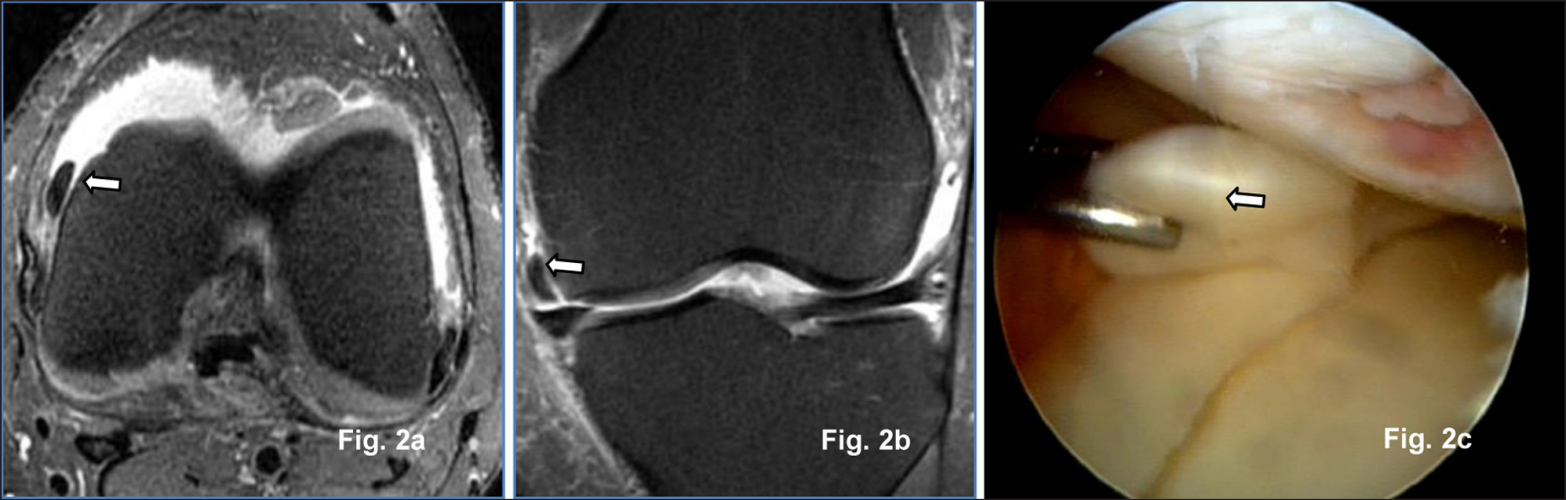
Transversal (a) and coronal PD Fat Sat (b) 1.5-T MR images: Medial meniscus unstable lesion in medial para-condylar recess (arrow) confirmed at arthroscopy (arrow) (c).
